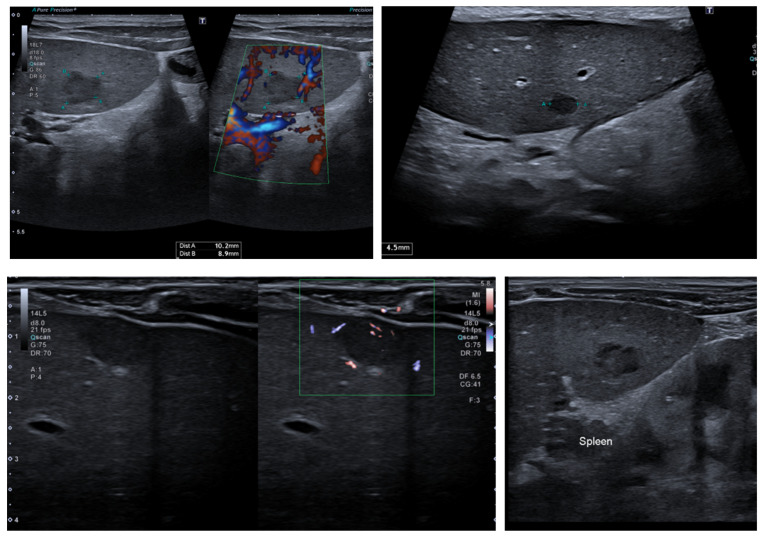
The ultrasound exam revealed three lesions on the liver and one on the spleen.
